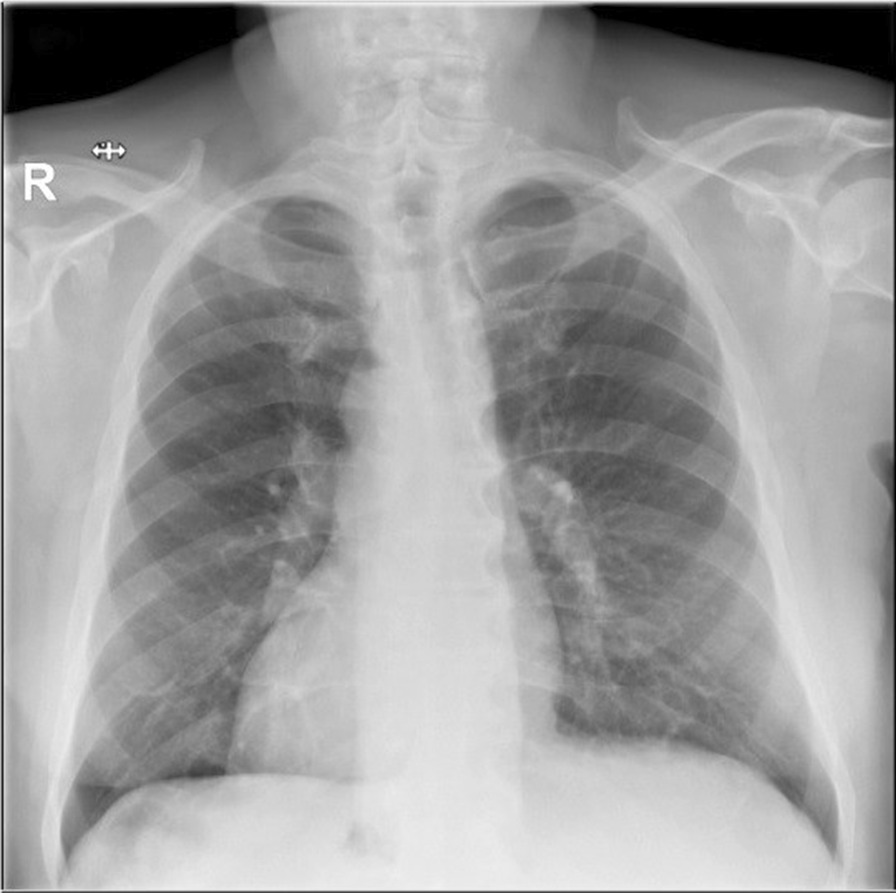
Chest X-ray of the patient with dextrocardia
